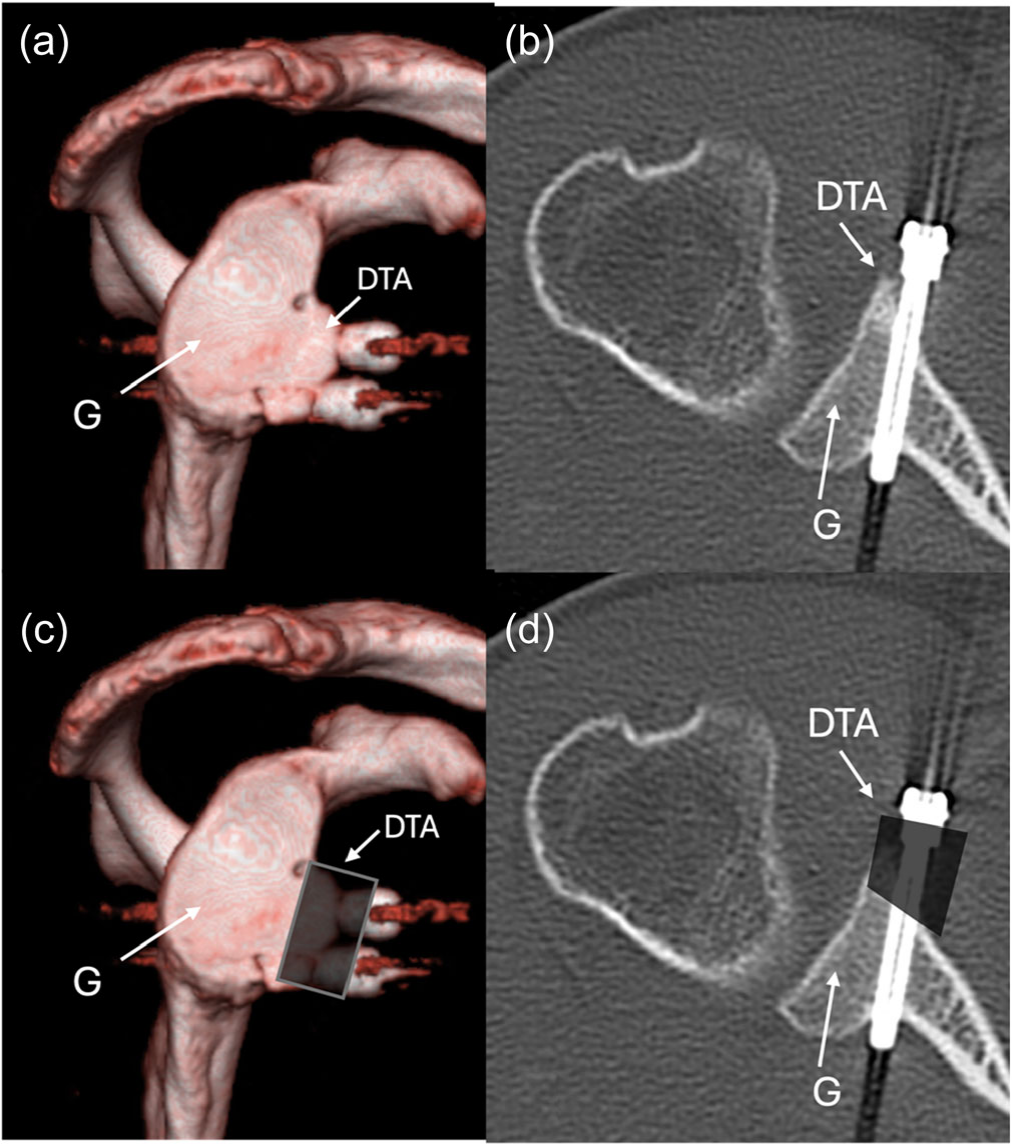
(a) Three‐dimensional computed tomography (CT) scan *en‐face* view. (b) Axial two‐dimensional CT scan view of a right shoulder demonstrating continuous cortical structure with the native glenoid. In (b) the anterior rim of the glenoid is in continuity in the axial plane, and the glenoid surface is curved and lacks discontinuity, often seen as sharp angles where the graft was inserted. (c) and (d) Estimation of where the graft would have been placed, to highlight its remodelling. DTA, distal tibia allograft; G, glenoid.
